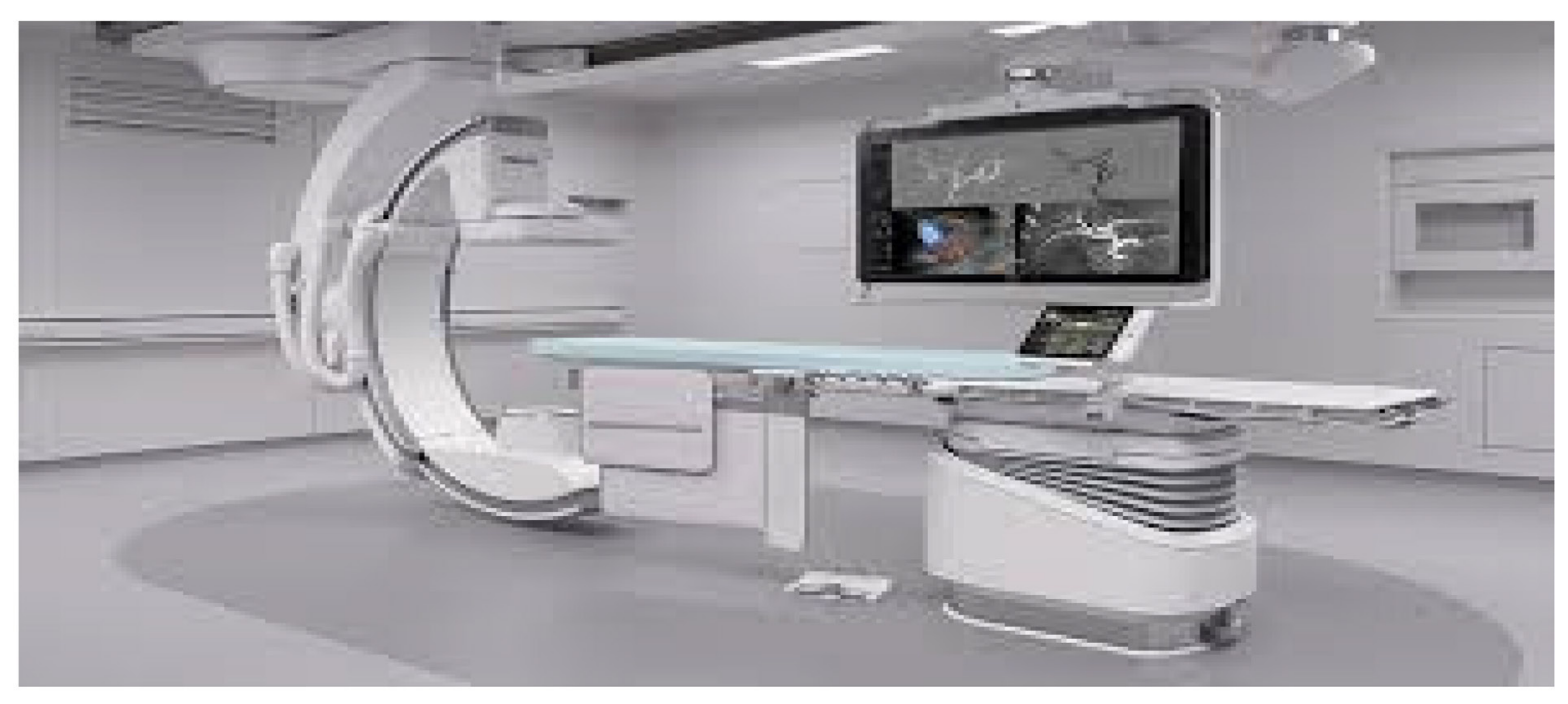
CBCT Azurion 7.1. (ceiling mounted) from Philips company (Koninklijke Philips N.V., Amsterdam, The Netherlands).
